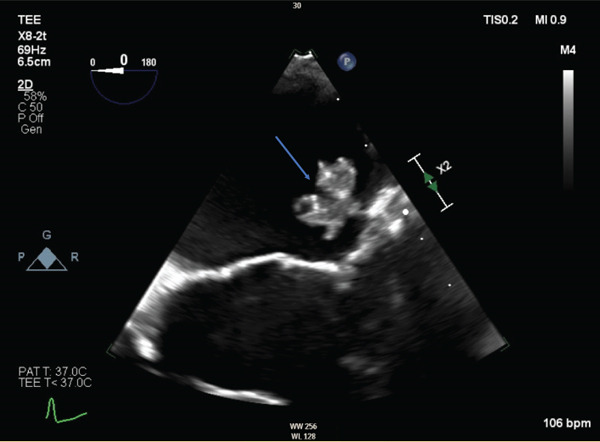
A transesophageal echocardiogram demonstrated a medium mobile mass measuring 1.43 × 0.805 cm on the mitral valve, with a dilated left atrium.
